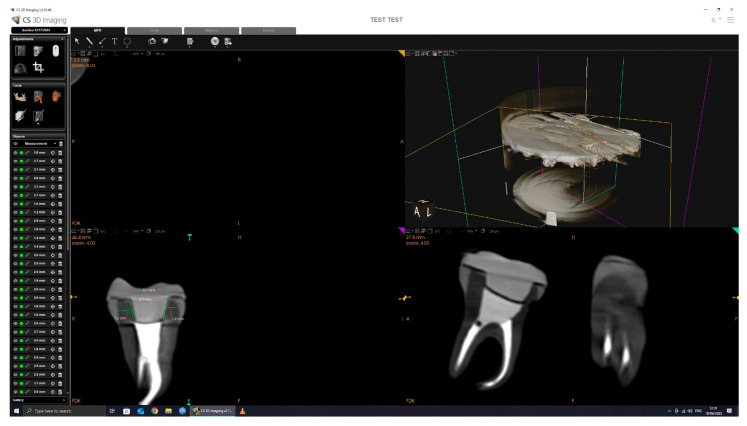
CBCT scan and measurement.
